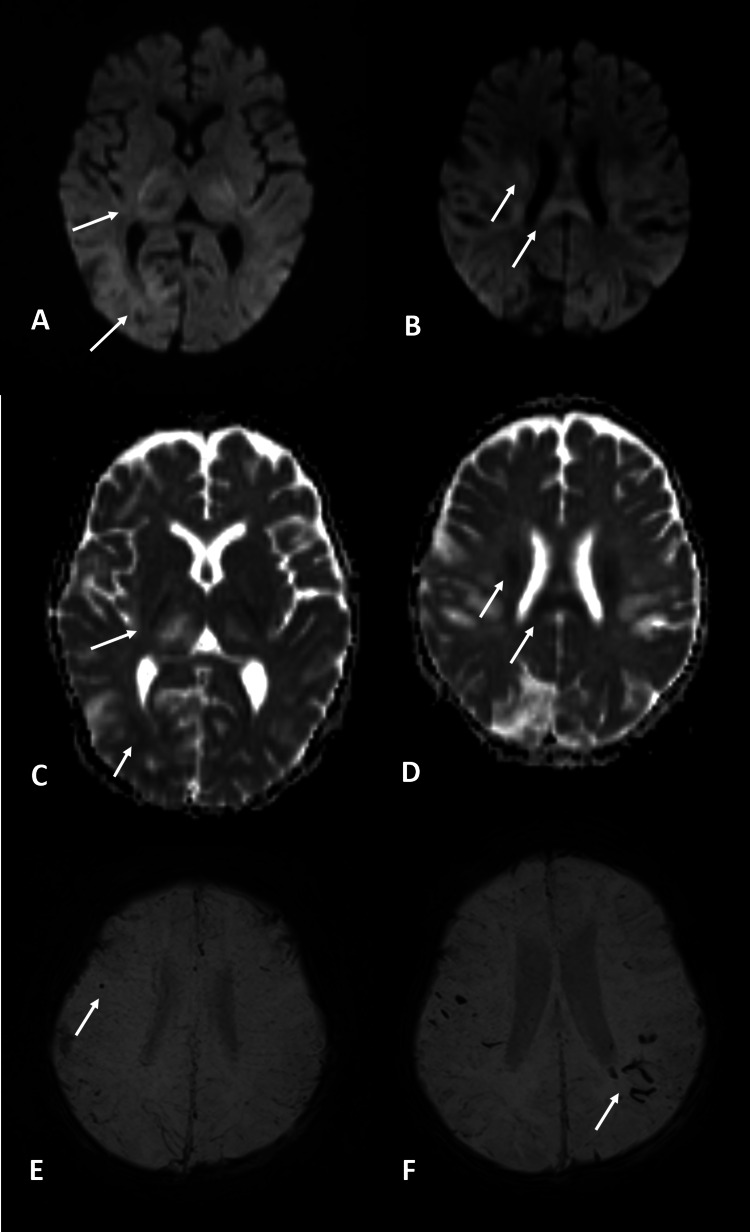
MRI – diffusion and SWI Diffusion (A, B) and ADC map (C, D) at the initial exam show restriction in the posterior limb of the internal capsule, cortico-subcortical junction of the occipital lobes (A and C), the posterior body of the corpus callosum, and corona radiate (B and D). A little dot of hemorrhage was visualized in the frontal lobe at the initial exam as a blooming artifact in the susceptibility-weighted image (E). In the follow-up exam, more hemorrhagic changes were visualized in the parietal lobes (F) MRI: magnetic resonance imaging; SWI: susceptibility-weighted imaging; ADC: apparent diffusion coefficient
